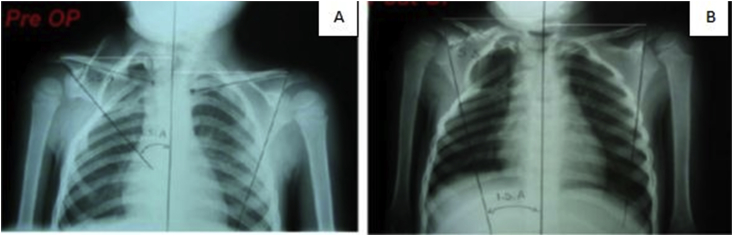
measurement method of upper and lower scapular angle in radiography. A. Preoperative B. Postoperative
